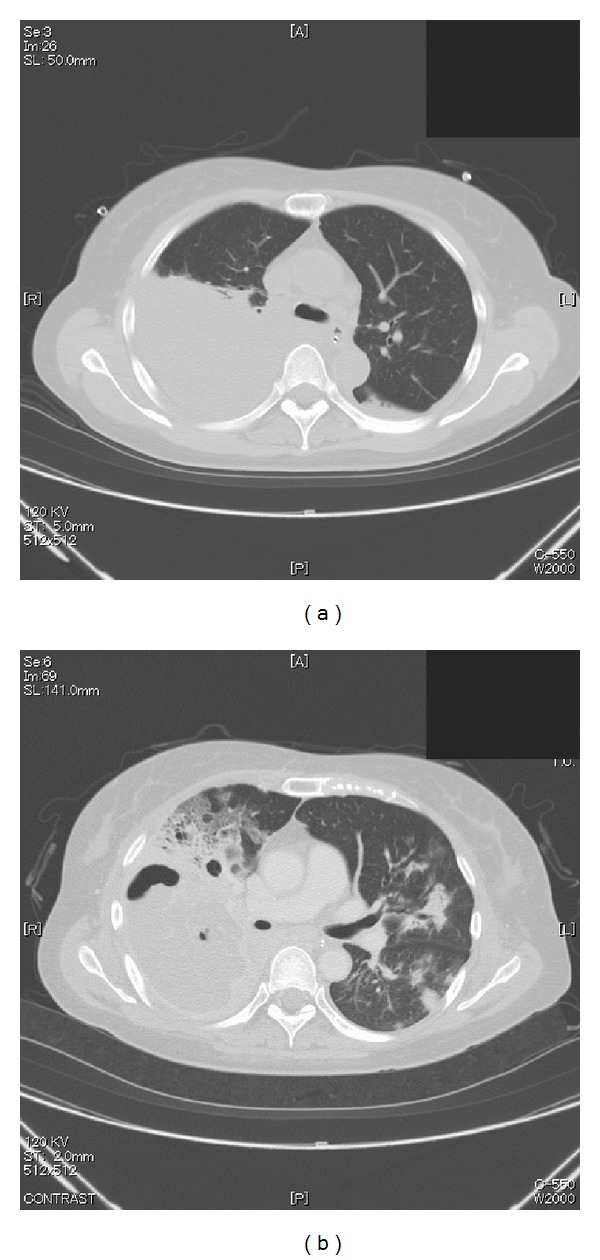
(a) Chest CT (POD 4). The scan shows a broad infiltrating shadow with air bronchogram mainly in the dorsal portion of the right lung, leading to a diagnosis of pulmonary suppuration. (b) Chest CT (POD 19). Two weeks later, the broad infiltrating shadow has expanded and shows storage of liquid. Both lungs have ground glass opacity and contain a maculate infiltrating shadow.
